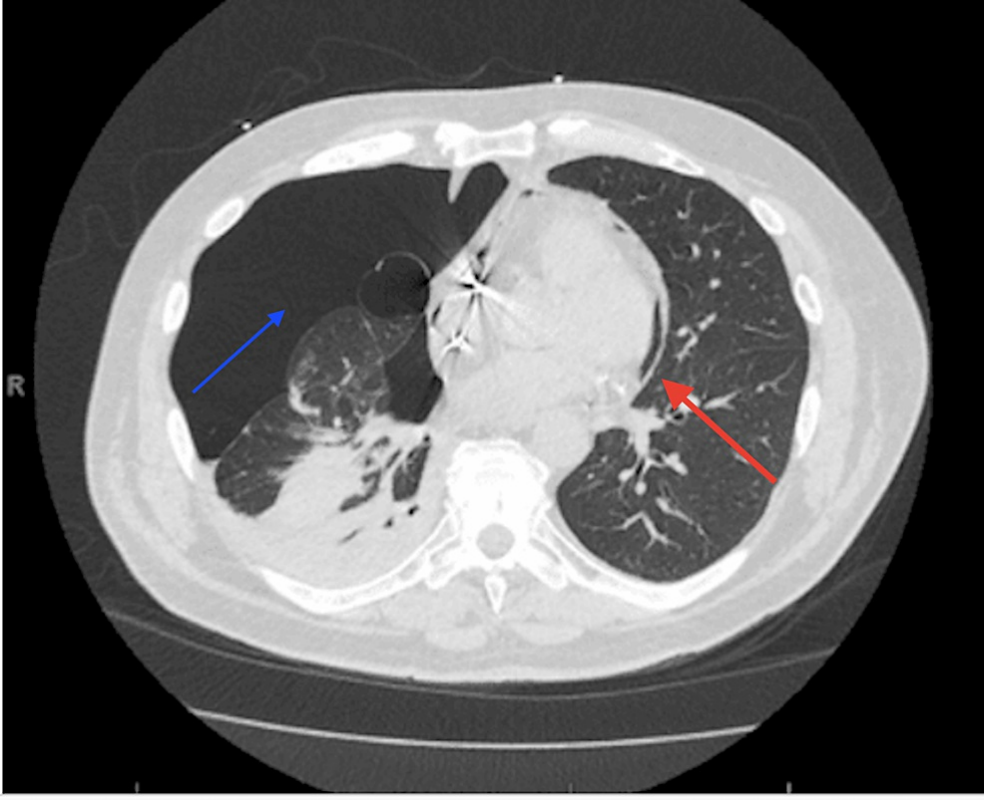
CT of the chest showing both a right-sided pneumothorax (blue arrow) and a contralateral pneumopericardium indicated by the red arrow.
